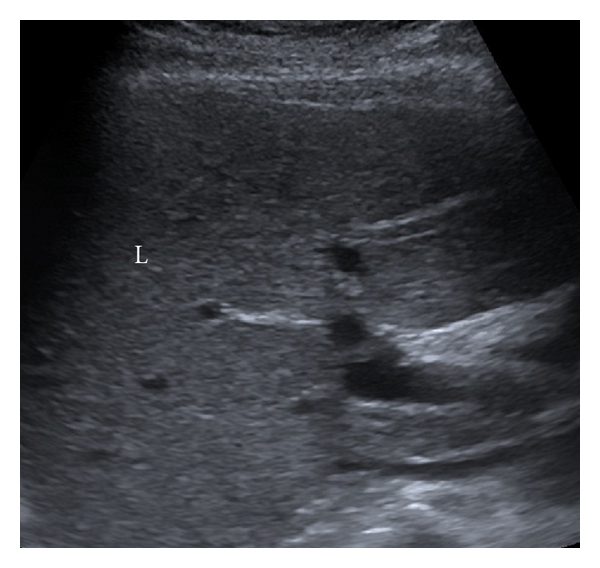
Ultrasonographic image of liver (L). No prominent lesion could be demonstrated.
